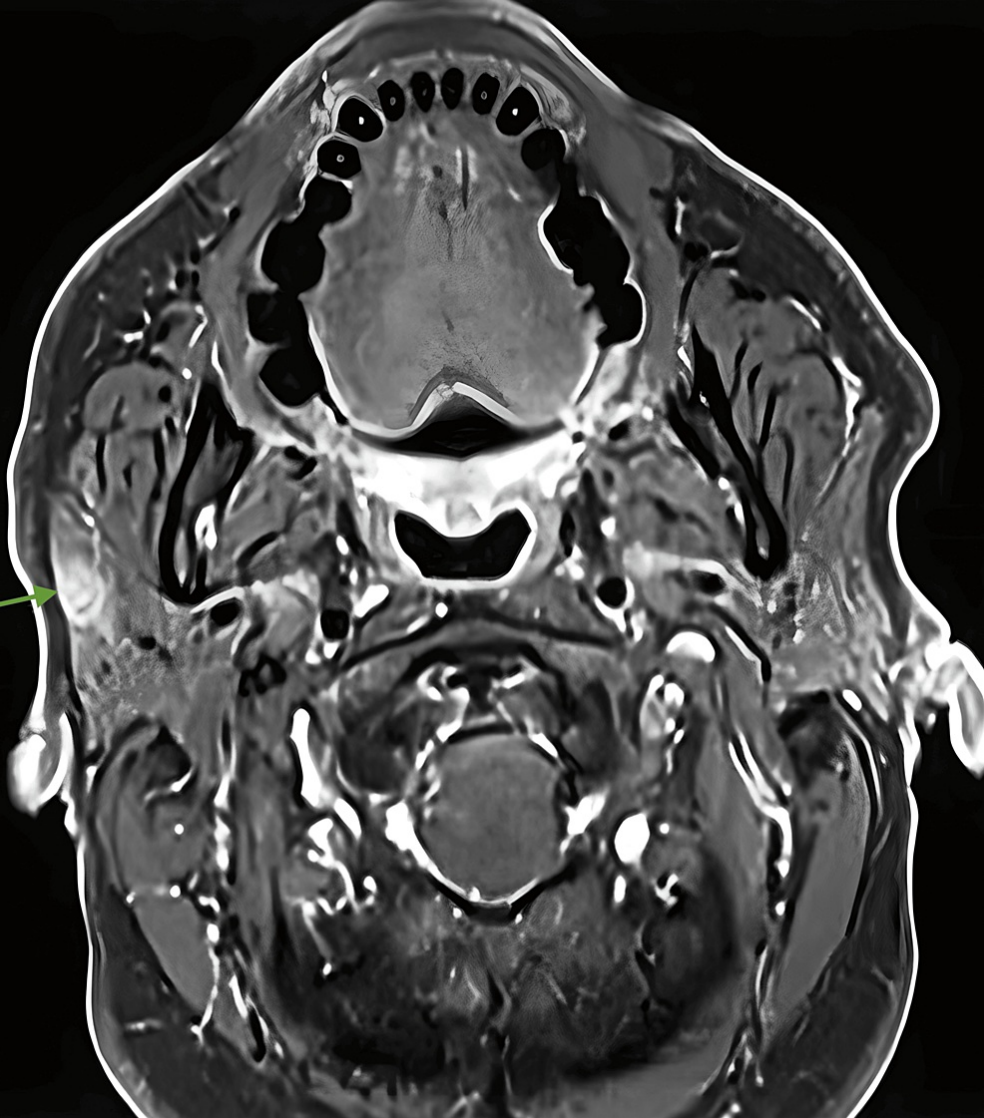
A MRI of the right parotid gland showing 10 mm × 5 mm indeterminant lentiform-shaped lesion
